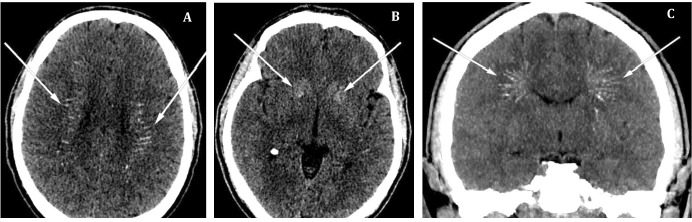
Patient brain CT scan. A, B – transverse plane images; C – coronal plane image. White arrows show intracerebral calcifications.
